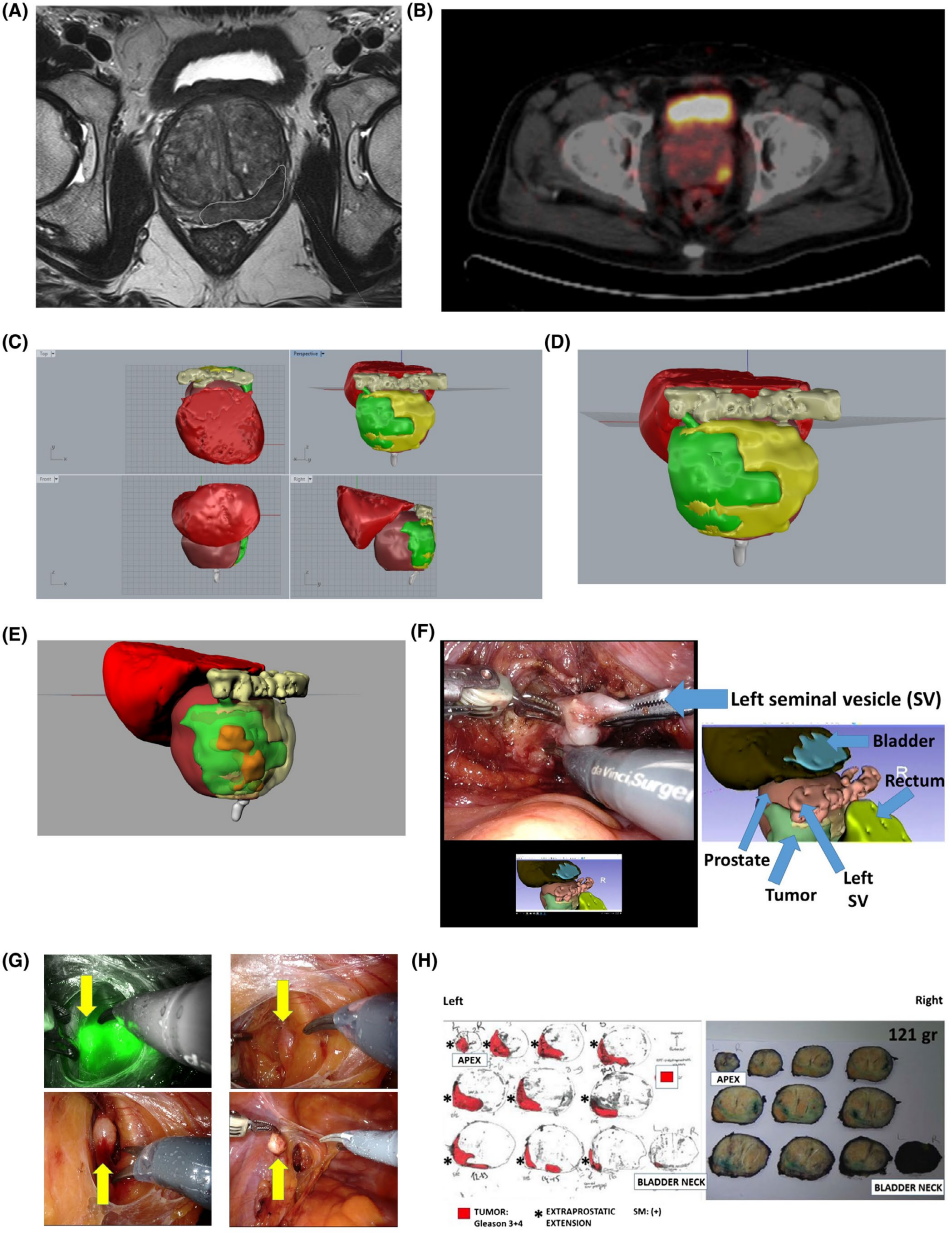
A, mpMRI image. Axial T2‐weighted space sequence shows a 3.5 cm PI‐RADS 5 lesion in the left posterior peripheral zone at the level of midgland‐basis. There is suspicion of left seminal vesicle and NVB involvement. Borders of the lesion which was pathologically known to be adenocarcinoma was drawn. B, Ga68 PSMA‐PET/CT: PSMA uptakes were seen in left lateral‐medial sides of mid‐gland and left medial side of apex with 3.21 SUVmax. C, 3D reconstructed image of the prostate. Left up (*yx* axis): appearance from the top, left down (*zx* axis): appearance from front, right down (*zy* axis): appearance from right, right up (*xyz* axis, perspective). Green: tumor on MRI, yellow: peripheral zone, purple: anterior‐transition zone, red: bladder. D, 3D reconstructed image of the prostate (maximized perspective, *xyz* axis). Green: tumor on MRI, yellow: peripheral zone, purple: anterior‐transition zone, red: bladder. Tumor involving almost the entire left half of the prostate gland with obvious extraprostatic extension is visible (green). E, 3D reconstructed image of the prostate with tumor on Ga68 PSMA‐PET/CT overlap in addition to mpMRI (maximized perspective, *xyz* axis). Orange: 68Ga‐PSMA uptake area, Green: tumor on MRI, yellow: peripheral zone, purple: anterior‐transition zone, red: bladder. F, Real time use of 3D reconstructed image of the prostate during RARP and intraoperative surgical appearance. Due to the possible involvement of left seminal vesicle and NVB by the tumor that also appears in the 3D images, console surgeon did not preserve left NVB and did a careful dissection at the level of left seminal vesicle. G, ICG guided pelvic LN dissection (left side). Please note ICG(+) LN that was excised and sent for intraoperative pathological frozen evaluation that was reported as metastatic. H, Postoperative pathology mapping of the prostate
